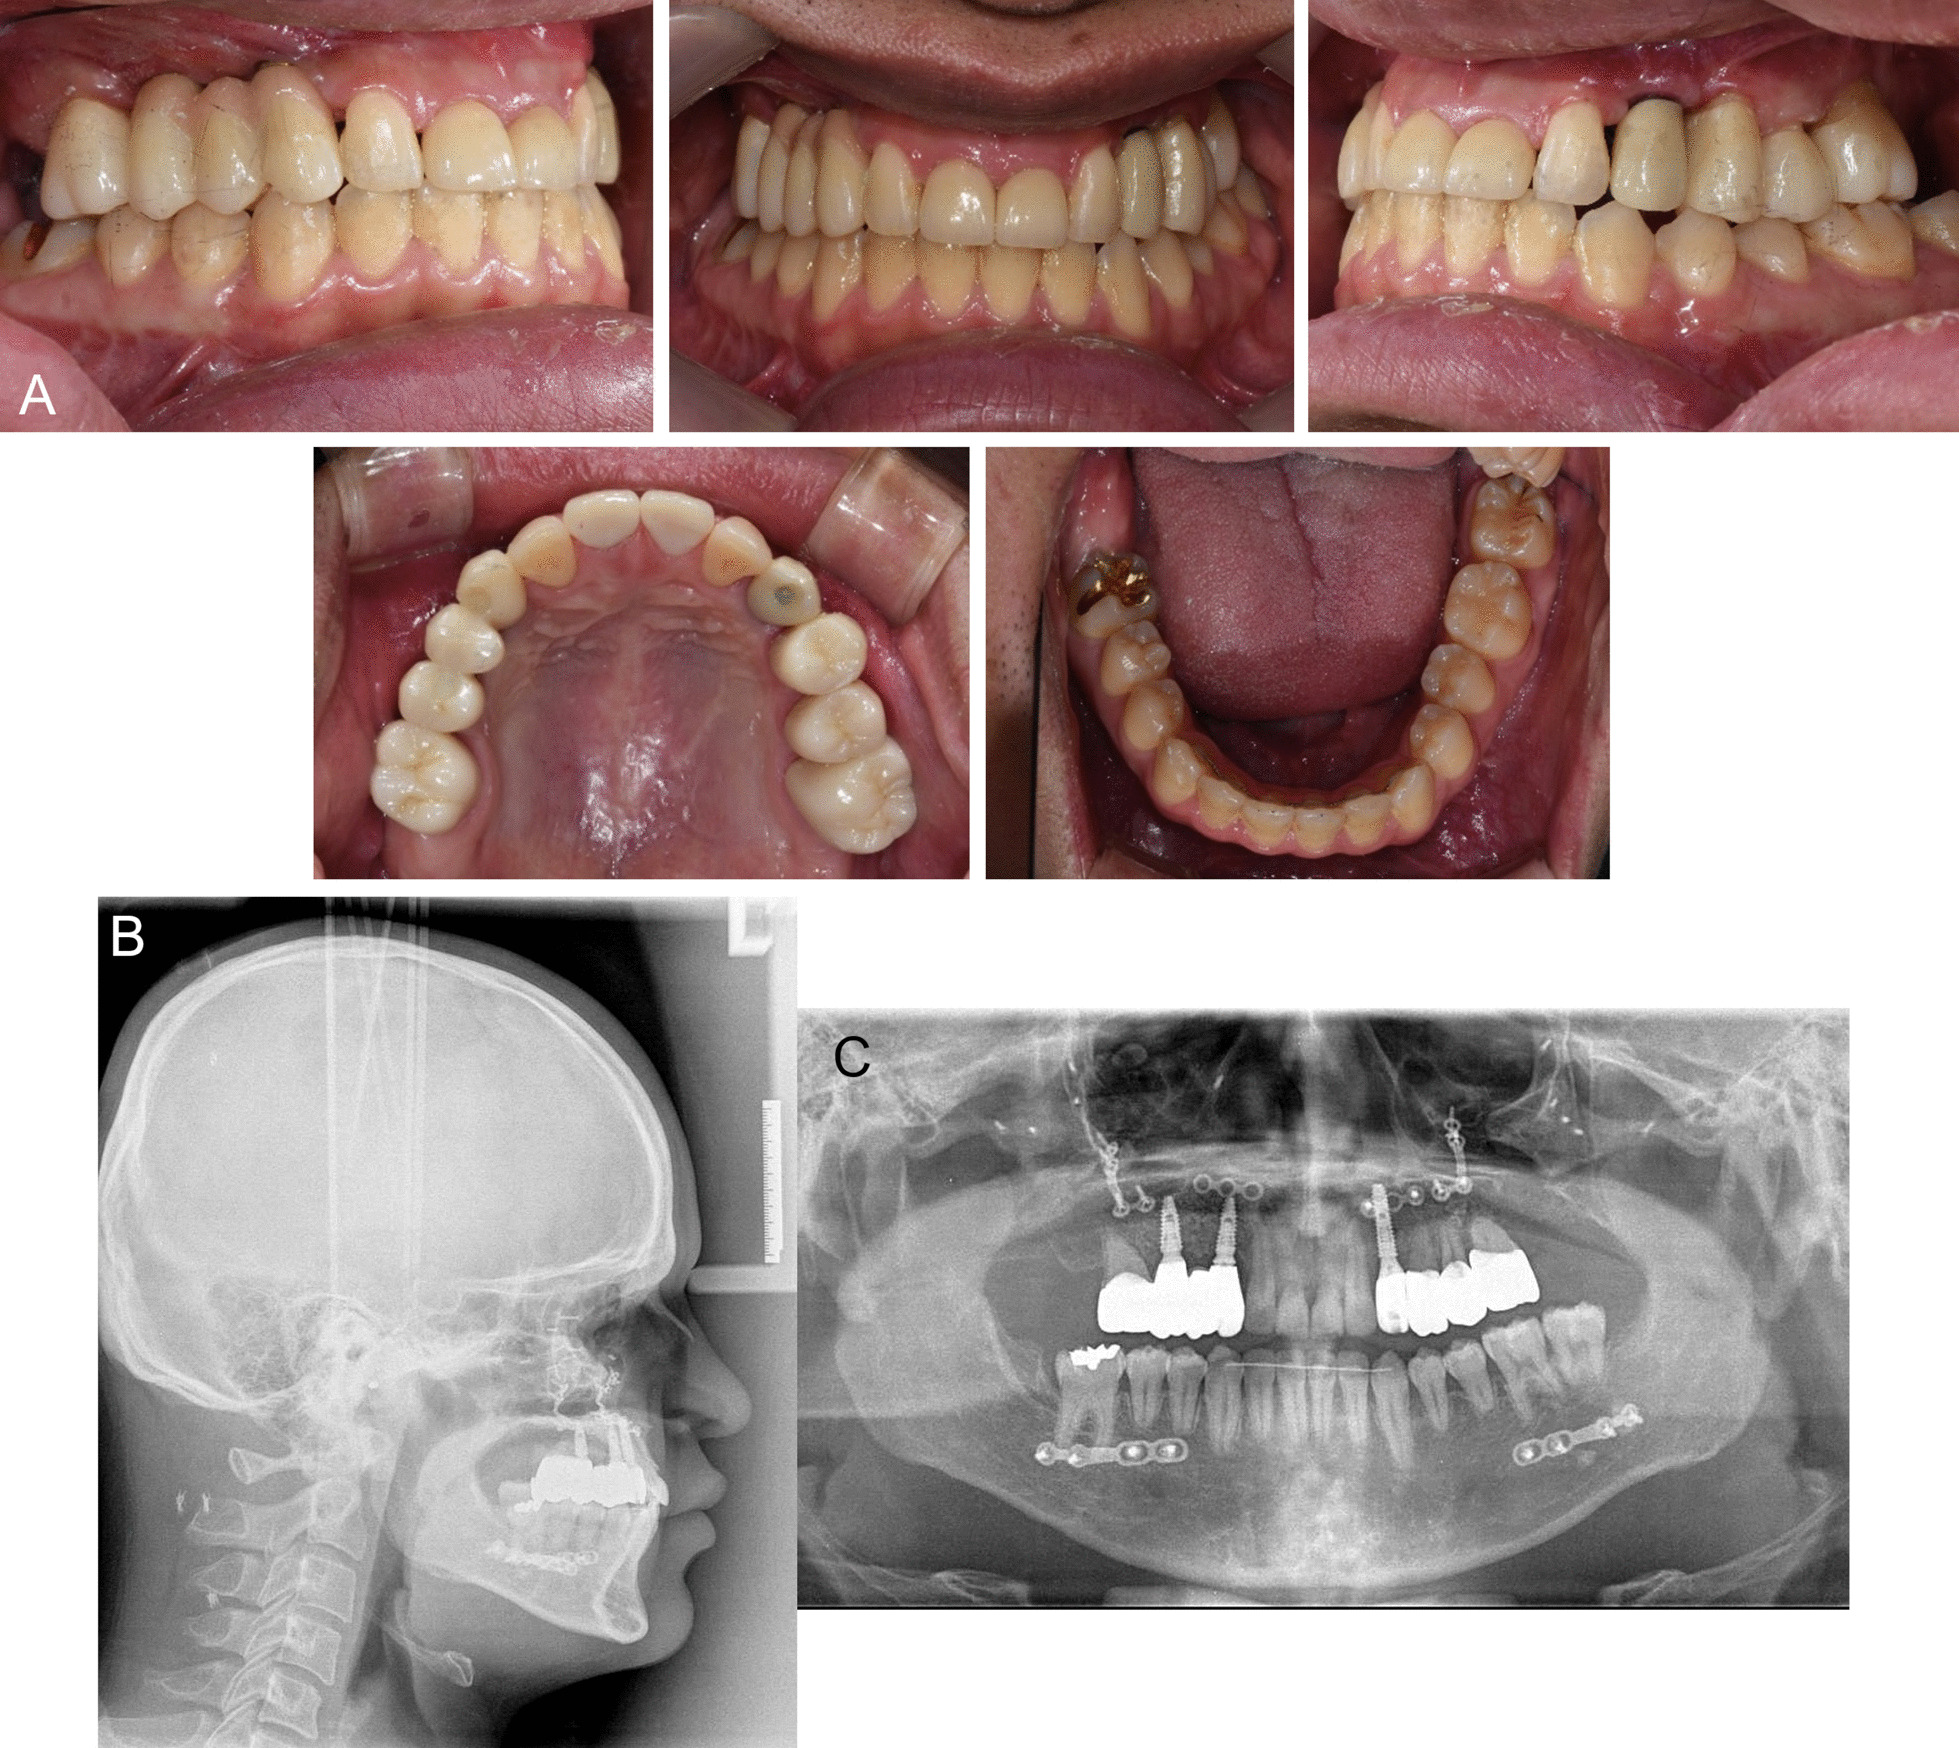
Posttreatment records of the patient. A. Intraoral photograph; B. Lateral cephalogram; C. Panoramic radiograph
